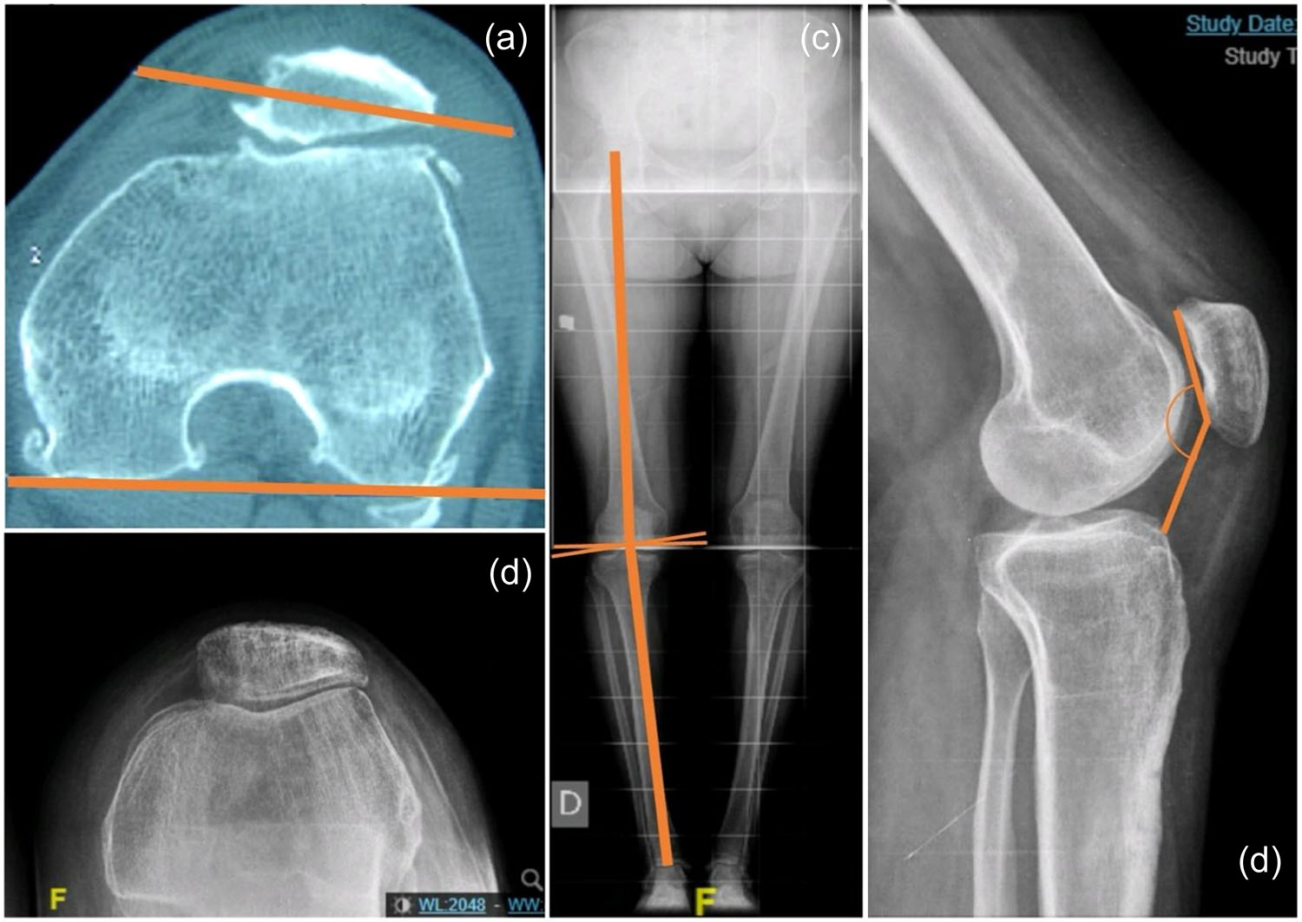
Radiological measurements. The four radiological projections used in the measurements: (a) axial view of the CT scan of the knee. Outlined in orange is the measurement of the patellar tilt angle. The tibial tubercle‐trochlear groove was also obtained. (b) Skyline view to assess patellar morphology and degree of patellofemoral osteoarthritis. (c) Full‐length lower limb x‐ray to assess mechanical femorotibial angle as outlined in orange. (d) Caton–Deschamps index in the lateral 30° view. Also, Dejour index is assessed in this projection. CT, computed tomography.
